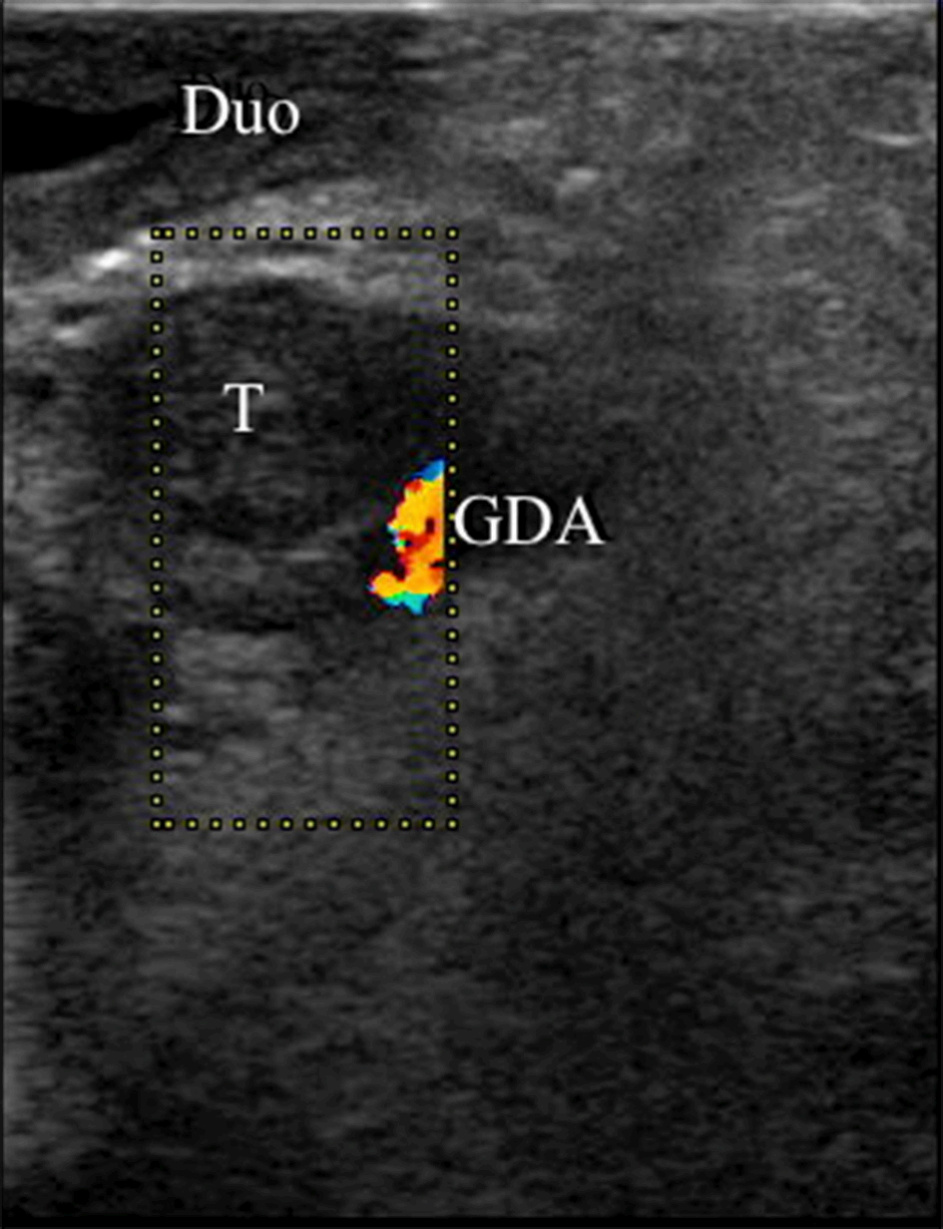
Intraoperative ultrasound of a focal CHI lesion. A nine mm hypo-echoic focal CHI lesion (“T”) is identified adjacent to the gastroduodenal artery (“GDA”) and the duodenum (“Duo”).
